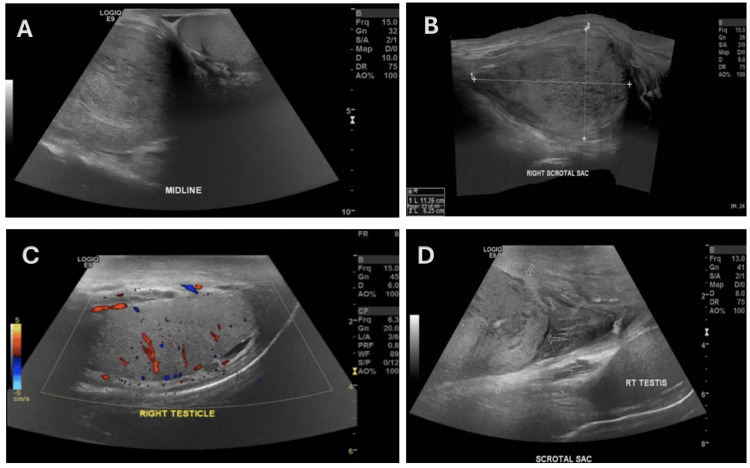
Scrotal ultrasound (A-B) Grayscale ultrasound images showing a large extratesticular lesion measuring 11 × 6 cm. (C) Doppler images demonstrating internal vascularity within the lesion. (D) Normal appearance of the right testes.
